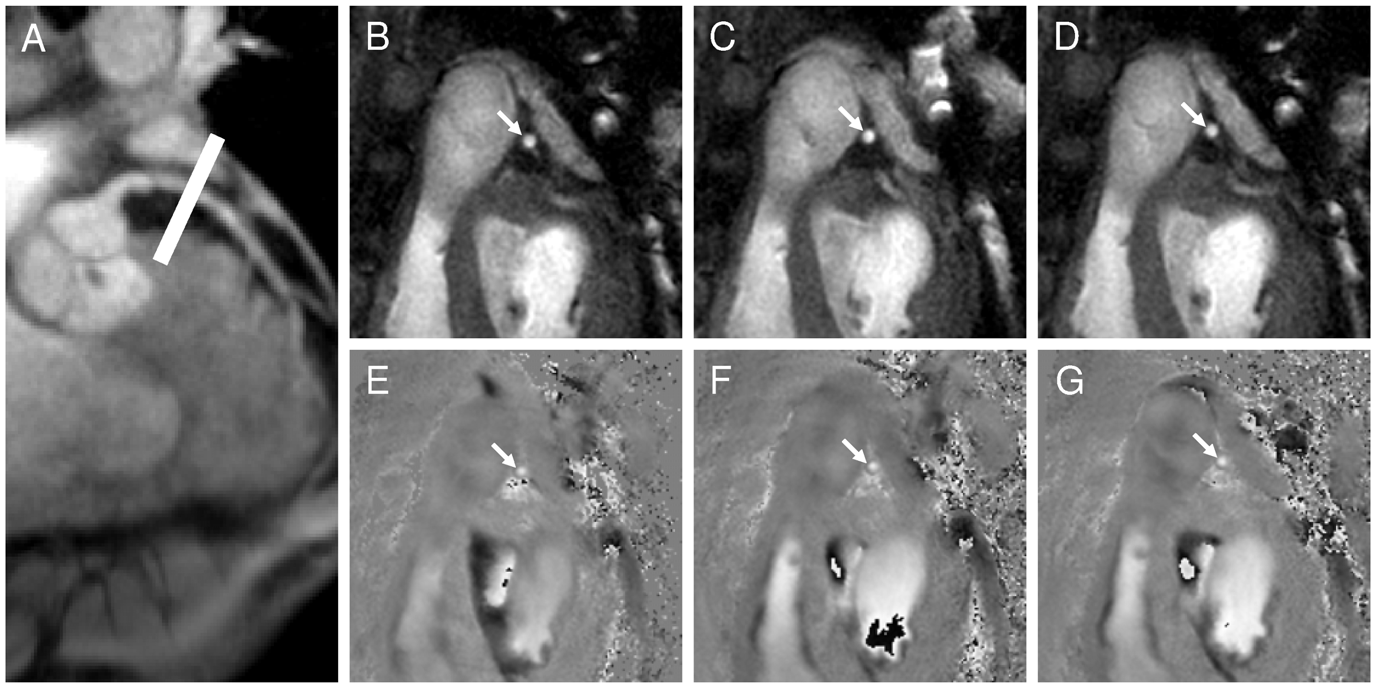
Typical anatomical and flow-velocity encoded coronary images using magnetic resonance imaging at rest and during sequential isometric handgrip stress in a CAD patient. A scout scan obtained parallel to the left anterior descending (LAD) artery (A) is shown together with the location for cross-sectional imaging (white line). The corresponding cross-section of the LAD is shown at rest (B) and during the first (C), and second handgrip stress (D, white arrows) and indicates no significant change in coronary cross sectional area during each stress. The white arrow in E shows a velocity-encoded image of the same LAD cross section at rest, during the first handgrip (F) and second handgrip stress (G). In this case, because the direction of blood flow is being analyzed in the LAD, the change in luminal coronary signal intensity (degree of “whiteness”) indicates a proportional change in through-plane coronary flow velocity.
